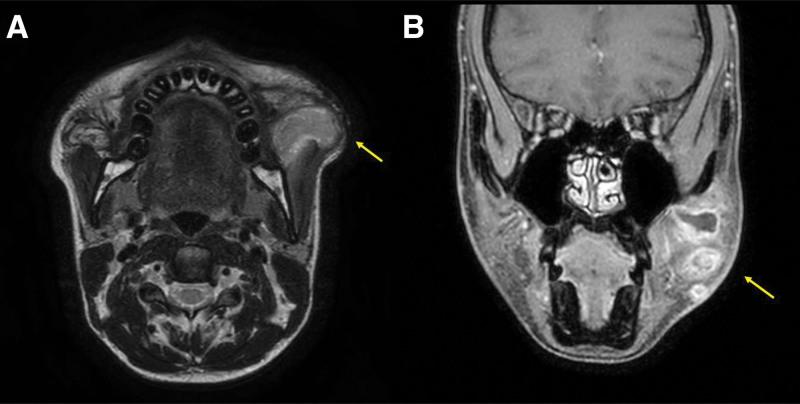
MRI revealing an infiltrative T2-hyperintense lesion (yellow arrow) beneath the SMAS (sub-SMAS) plane, with extension into the masseter muscle. (A) Axial view and (B) Coronal view. MRI = magnetic resonance imaging, SMAS = superficial musculoaponeurotic system.
